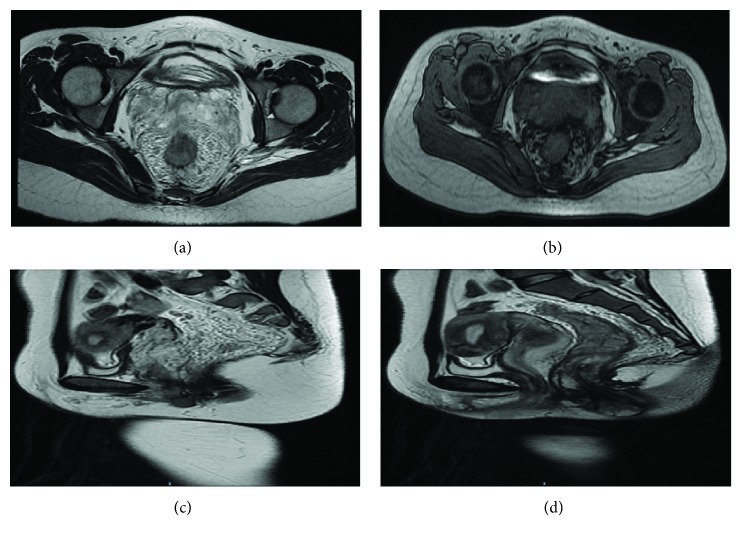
MRI findings of DCHR. (a) Thickened rectal wall and serpentine vessel. (b) Enlarged and serpentine vessel. (c) Serpentine vessel. (d) Thickened rectal and anal canal wall.
